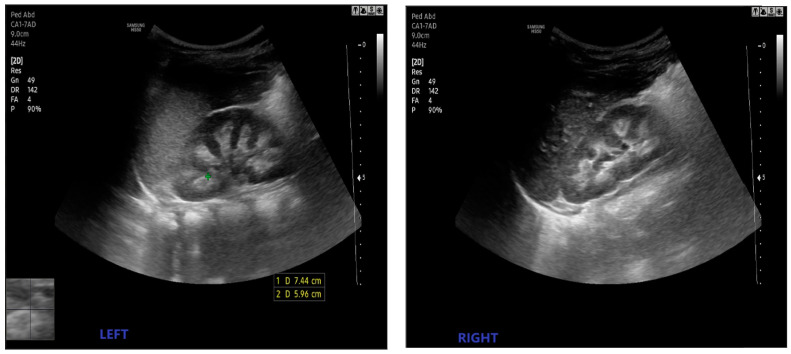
Case 1: ultrasound evaluation of the kidneys, showing the bilateral increased echogenicity of the renal pyramids (left, right).
